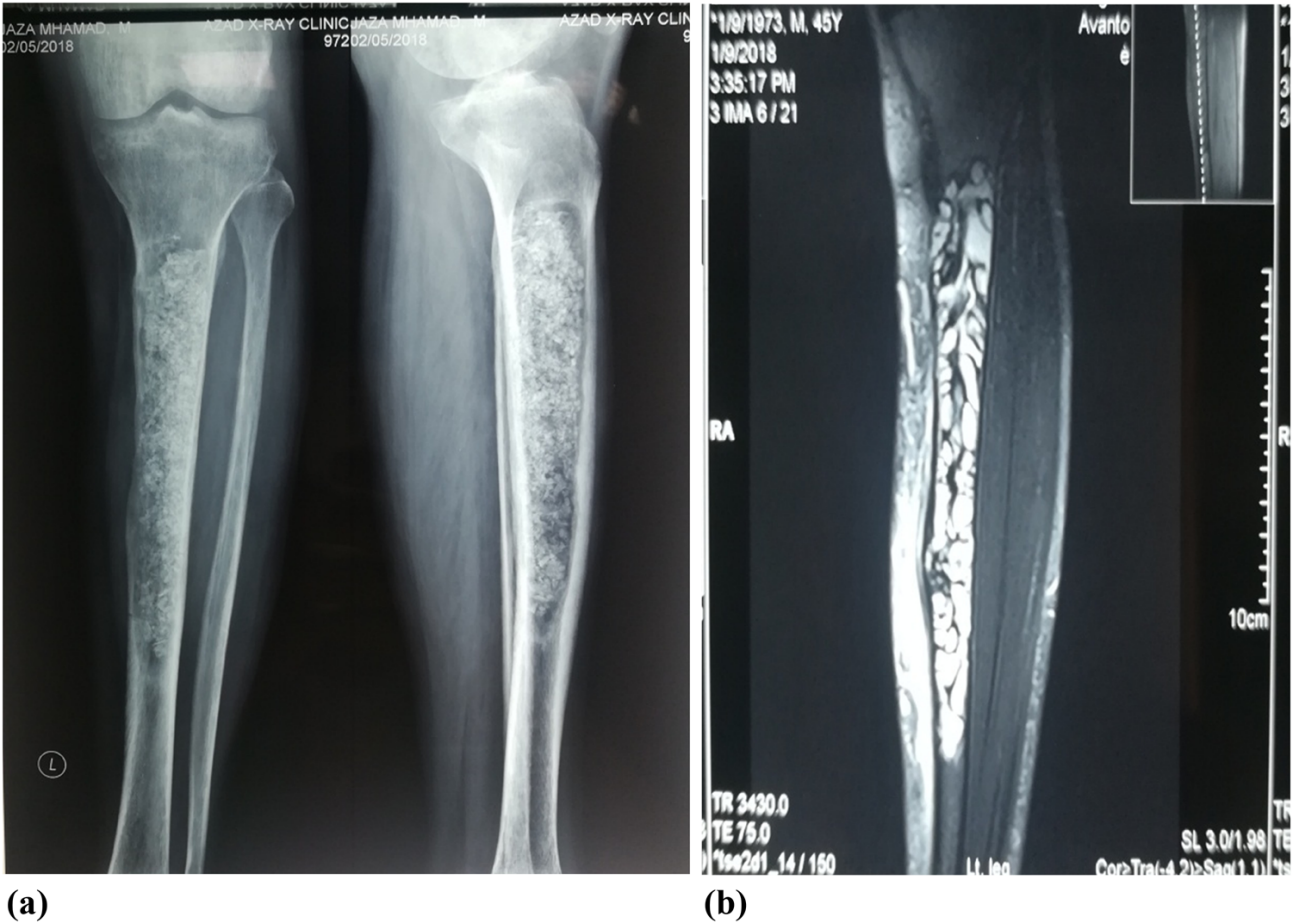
(a) A radiogram of tibial bone shows intramedullary, well-defined, diaphyseal, mixed lucent with multiple opaque shadows, mild bone expansion, no periosteal reaction, or cortical breach. (b) Coronal T2 fat suppression MRI sequence shows multiloculated cystic intramedullary diaphyseal lesion, no periosteal reaction or soft tissue component, no marrow oedema, and no fluid level. The photos are taken from patient number 5.
